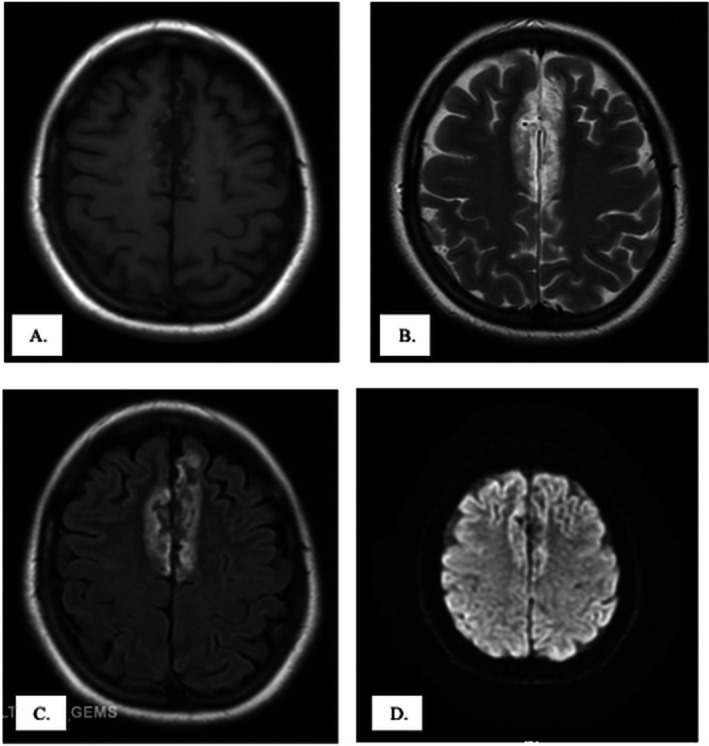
MRI of the brain showing bilateral frontal lobe lesions consistent with Bifrontal Encephalomalacia in different MRI sequences. T1‐weighted image (A) and Diffusion‐Weighted image (DWI, D) show hypointense bifrontal focal lesions. T2‐weighted image (B) and Fluid‐attenuated inversion recovery image (FLAIR, C) both show hyperintense bifrontal focal lesions. These findings reflect chronic post‐traumatic structural brain damage, which served as the epileptogenic substrate for the patient's delayed‐onset seizures. Identifying such lesions is critical in patients with remote head trauma, as they may predict long‐term risk of post‐traumatic epilepsy.
